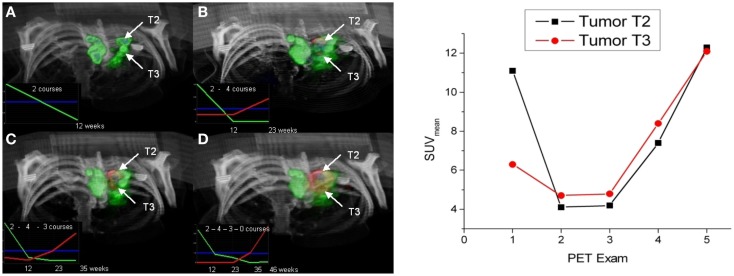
Left: 3D visualization of two tumors (T2 and T3) using parametric imaging with three basis functions: stable (blue), decreasing (green), and increasing (red) represented in the left corner of each image (the number of chemotherapy courses between each PET exam is mentioned). Parametric imaging using 2 (A), 3 (B), 4 (C), and 5 (D) exams. Right: SUV_mean_ evolution (calculated within a ROI defined by 40% of SUV_max_) for the two tumors. Note that the non-responding T2 tumor was detected with parametric imaging earlier (exam 2) than applying EORTC criteria that concluded to a stable disease between exam 2 and 3. Reprinted by permission of Necib (Ph.D Thesis).
